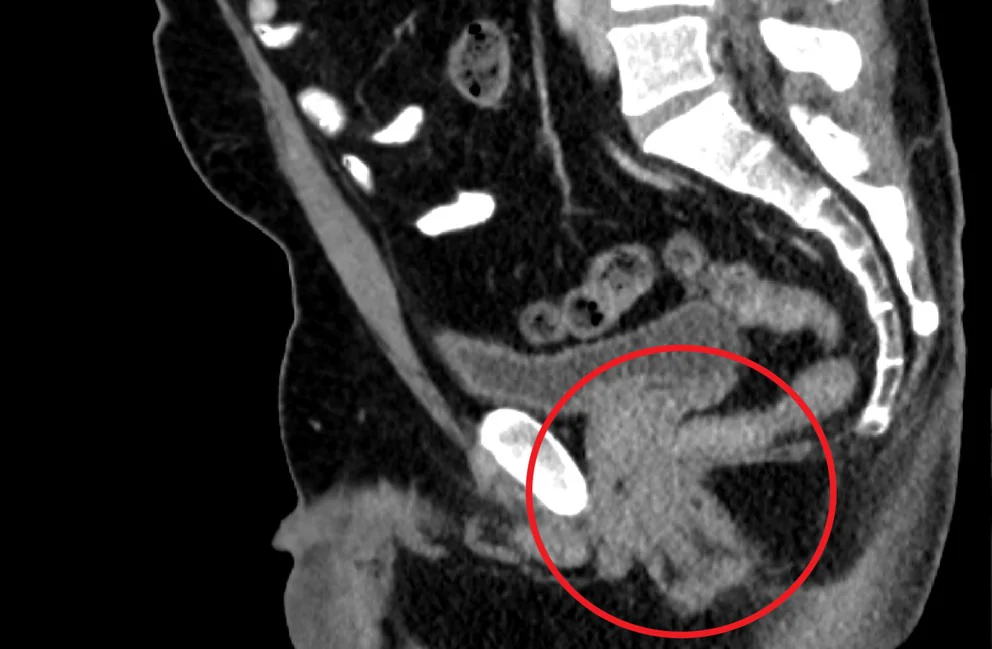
Abdominal CT scan with contrast (sagittal view) demonstrating diffuse rectal thickening (circled).
